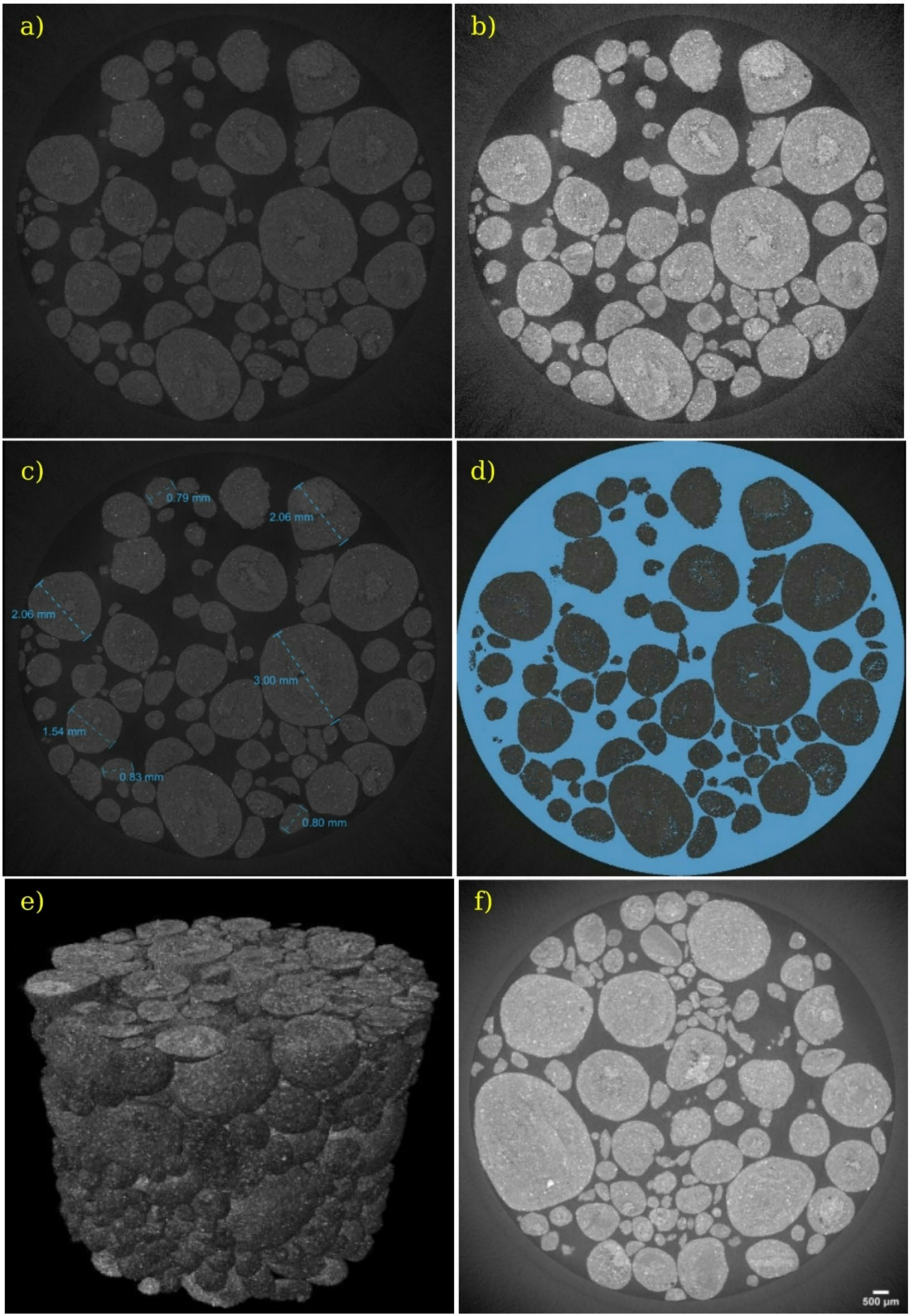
CT scan images of F-Slag sand a) original image, b) brightness adjusted, c) various particle sizes, d) Otsu segmented image, e) 3D rendering top view, and f) 3D rendering bottom view of the axial plane.
